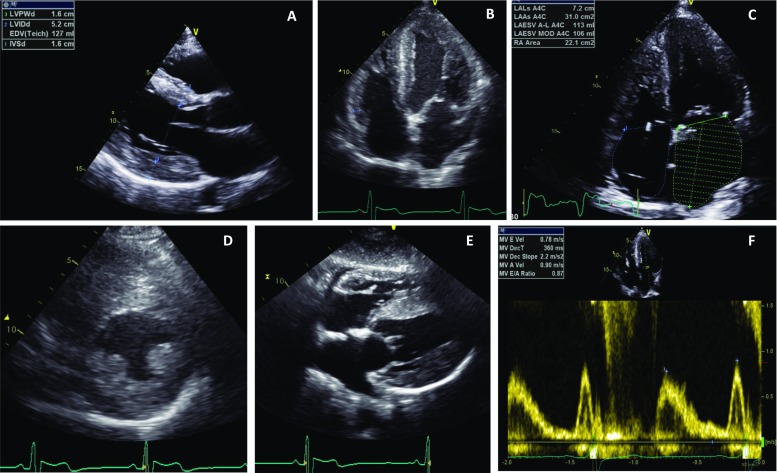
(Echocardiogram features of Anderson-Fabry disease). a Transthoracic echocardiogram (TTE) parasternal long-axis view (PLAX) demonstrating concentric LVH with an interventricular septum (IVS) and left ventricular posterior wall (LVPW) diameter of 16 mm. b Apical 4 chamber view on TTE demonstrating the presence of concentric LVH. c Moderately dilated left atrium (31 cm^2^) on apical 4-chamber TTE view. d TTE parasternal short-axis view (PSAX) at mid-ventricular level demonstrating concentric LVH and hypertrophied papillary muscles. e TTE subcostal view demonstrating concentric LVH. f Pulsed wave Doppler across mitral valve inflow demonstrating impaired relaxation (grade 1 diastolic dysfunction) with E:A ratio of 0.87
